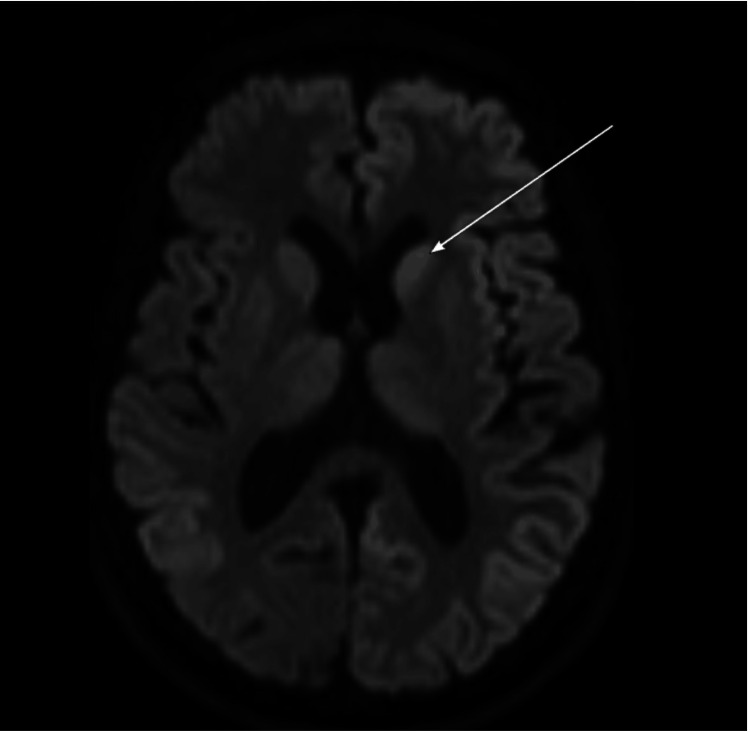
Diffusion-weighted imaging (DWI) showing diffusion restriction of the left caudate head (long arrow).
